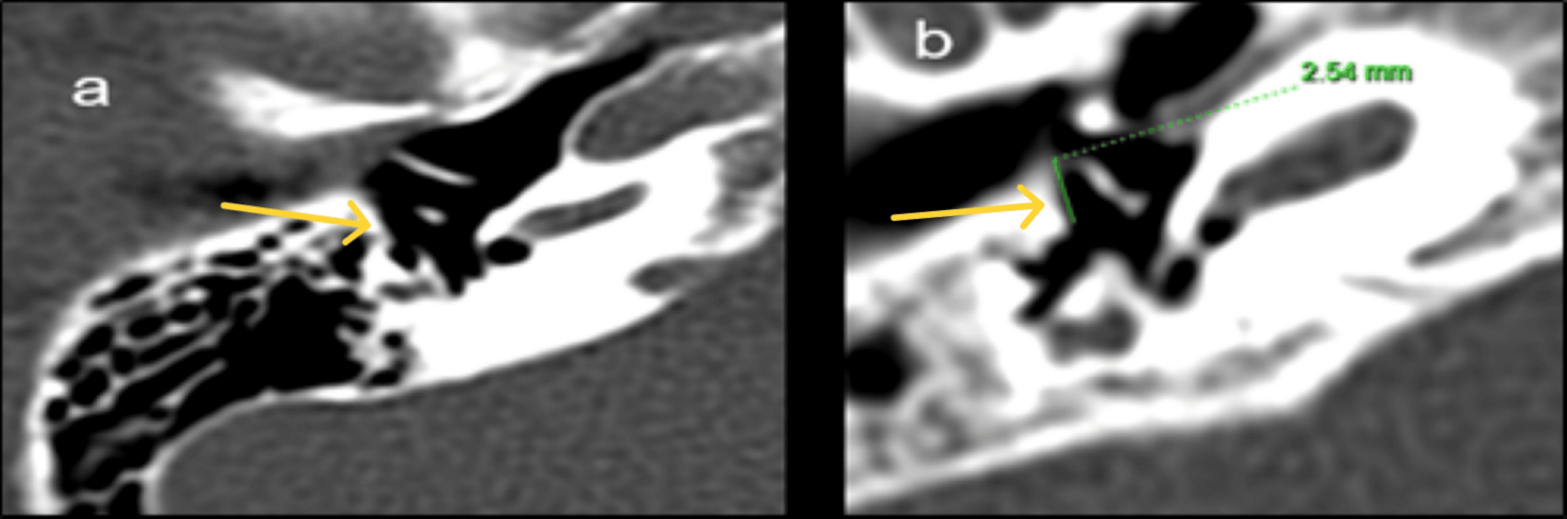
(a) and (b) Axial images of HRCT temporal bone demonstrating facial recess anatomy. (a) Normal and (b) narrow <3 mm. HRCT: high-resolution computed tomography.
